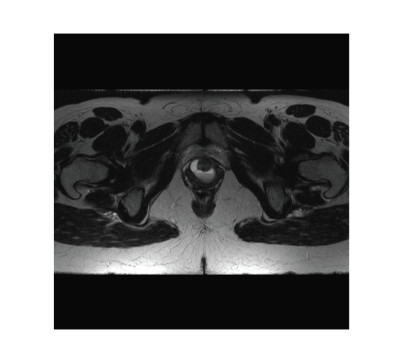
Urethral diverticulum on MRI (T2 weighted, fast spin echo). Patient presented with dyspareunia, urinary urgency and a normal physical exam. VCUG showed no evidence of urethral diverticulum. MRI revealed the correct diagnosis.
